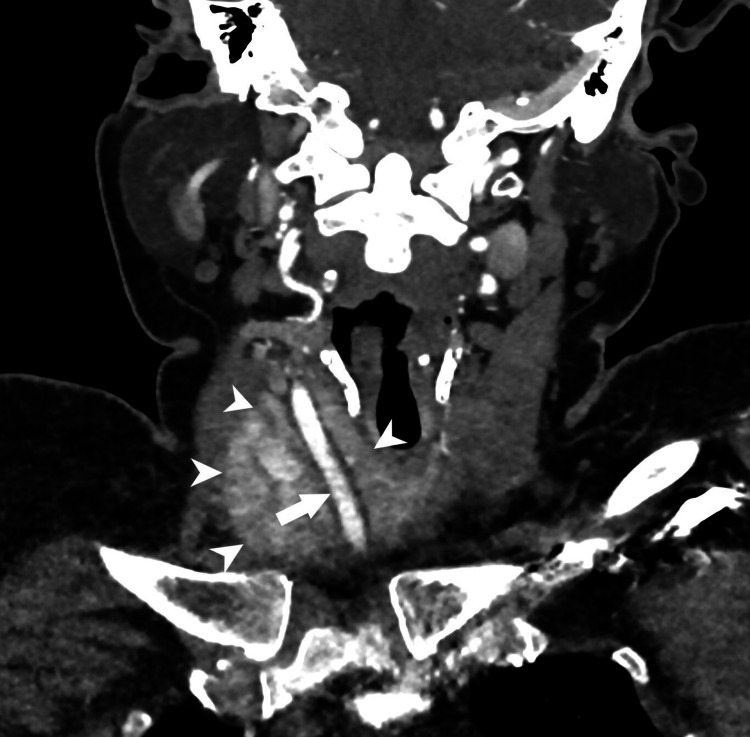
CT angiogram demonstrating large centrally necrotic nodal mass encasing right common carotid artery.
